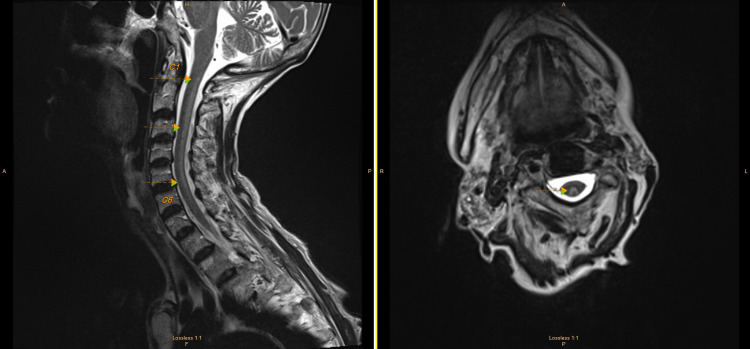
MRI cervical spine Sagittal T2WI (left) shows increased signal in the dorsal aspect of the spinal cord spanning C1-C6 with corresponding signal change on axial T2WI (right), often described as an inverted "v." T2WI: T2-weighted imaging; C1: first cervical vertebra; C6: sixth cervical vertebra
